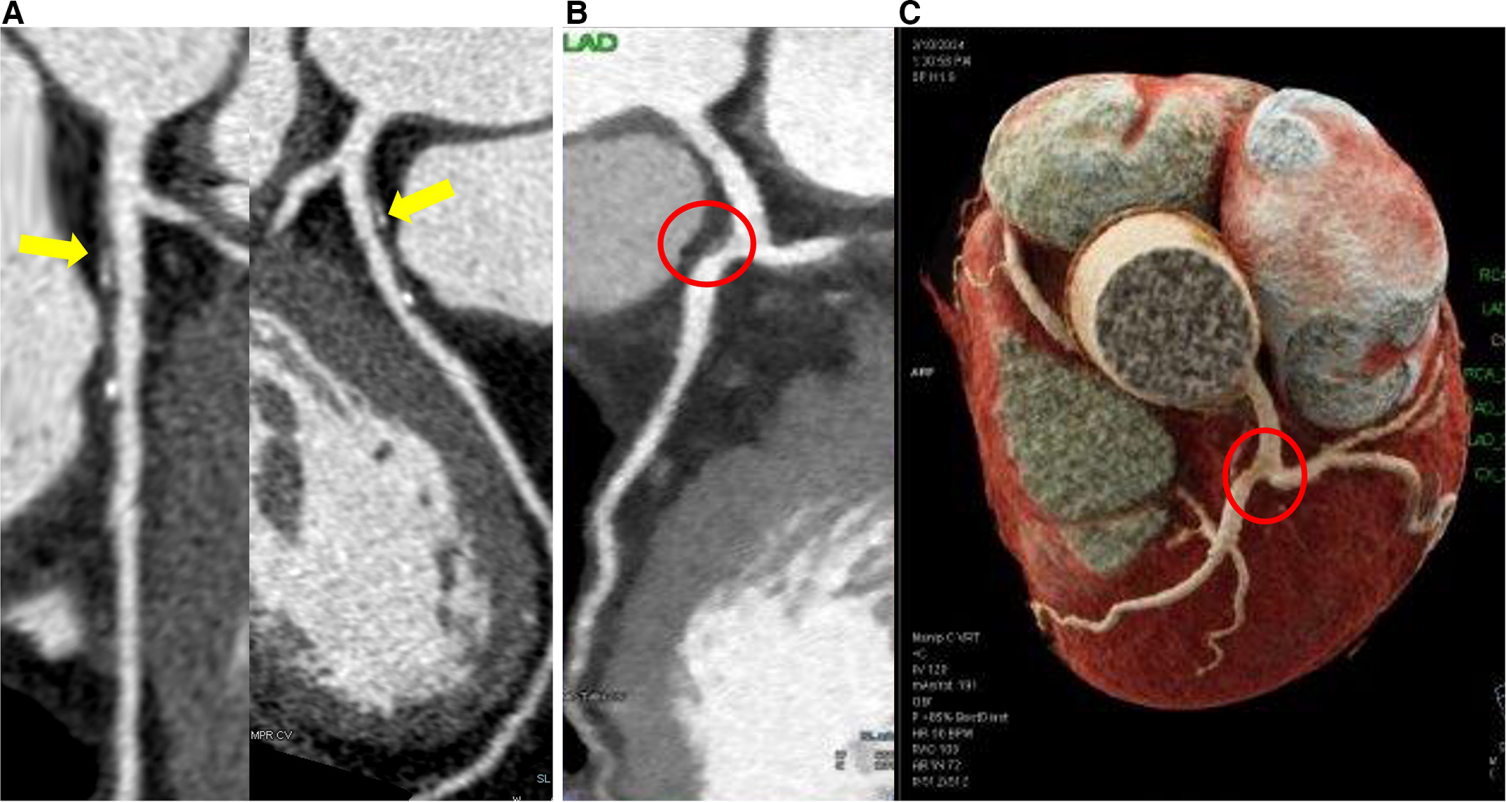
Coronary computed tomography angiography shows plaques located at the lateral wall of the bifurcation. (A) Eccentric partially calcified plaque in the left anterior descending coronary artery immediately downstream of the left main bifurcation. (B) Eccentric vulnerable plaque in lateral wall of left anterior descending coronary artery immediately downstream of the left main bifurcation (red circle) in 2D and 3D reconstruction (C).
